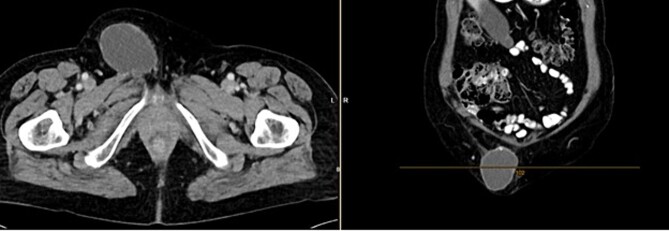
CT of the abdomen from 1 August 2022: size-progressive formation within the right inguinal hernia compared with the previous examination from 25 February 2022; no indication of tumor recurrence or metastasis-suspect lesions intra-abdominally, post-hysterectomy.
